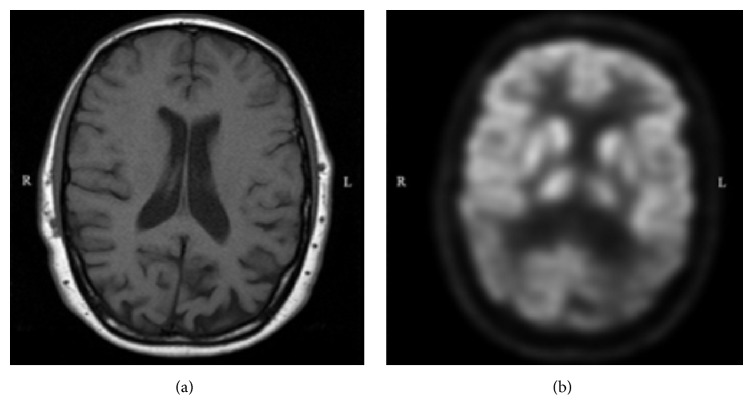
(a) T1 axial magnetic resonance imaging of brain shows predominant cerebral atrophy in the parietal lobes; (b) fluorodeoxyglucose positron emission tomography axial view showing bilateral parietal hypometabolism and hypometabolism of the posterior cingulate cortex.
